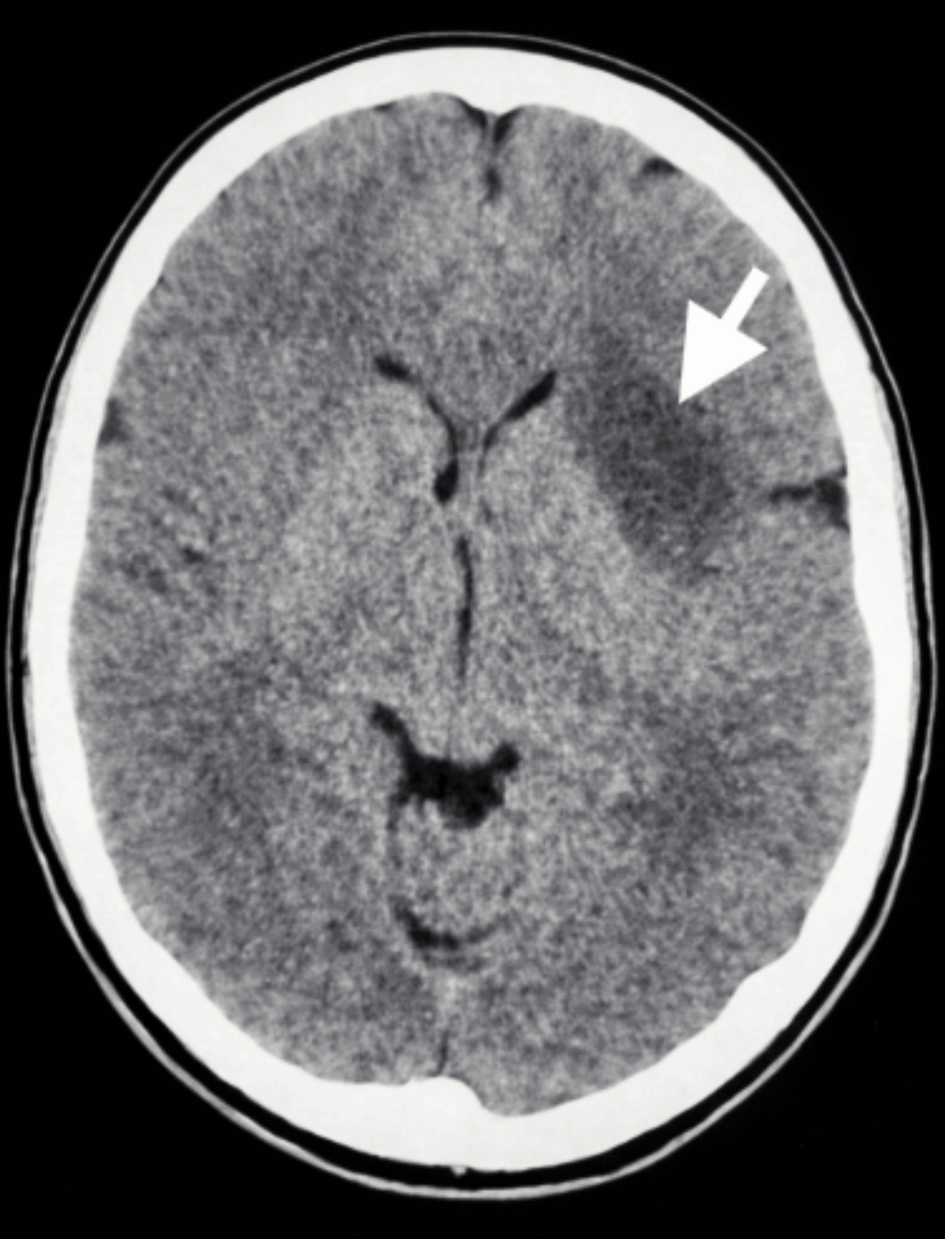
Axial non-contrast CT image of the brain. The white arrow indicates an area of low density seen in the left anterior aspect of the external capsule that extended to the anterior insular cortex and frontal white matter. This is consistent with a low-attenuation lesion suggestive of a low-grade tumor CT: computed tomography
